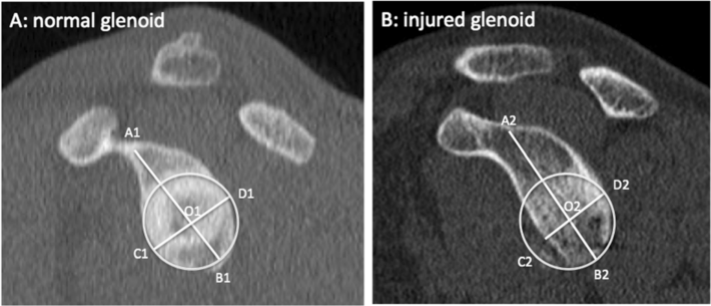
Glenoid Index. The Glenoid Index is calculated from injured width/normal width. Chuang *et al.* use the parameters of the normal glenoid to normalize the pre-injury glenoid width accounting for any height difference between shoulders. They then compare the ratio of post-injury width to pre-injury width. Although demonstrated here using 2D CT, the description of the Glenoid Index by Chuang *et al.* involves 3DCT. (Adapted from Chuang *et al.* [68])
